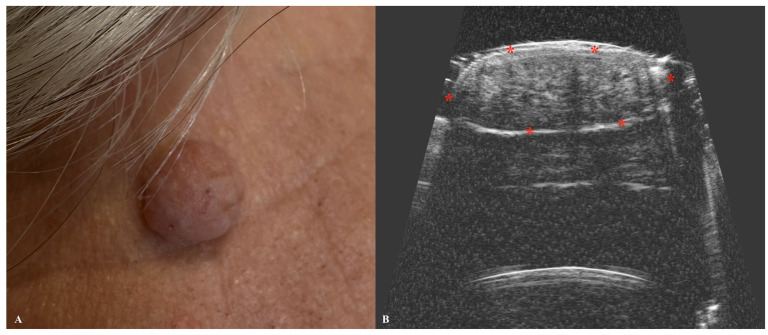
Macroscopic view of a forehead fibroma (A) with a corresponding ultrasonographic image (B), showing a well-defined tumor with mixed echogenicity and a hyperechoic surrounding band. Stars indicate tumor margins.
